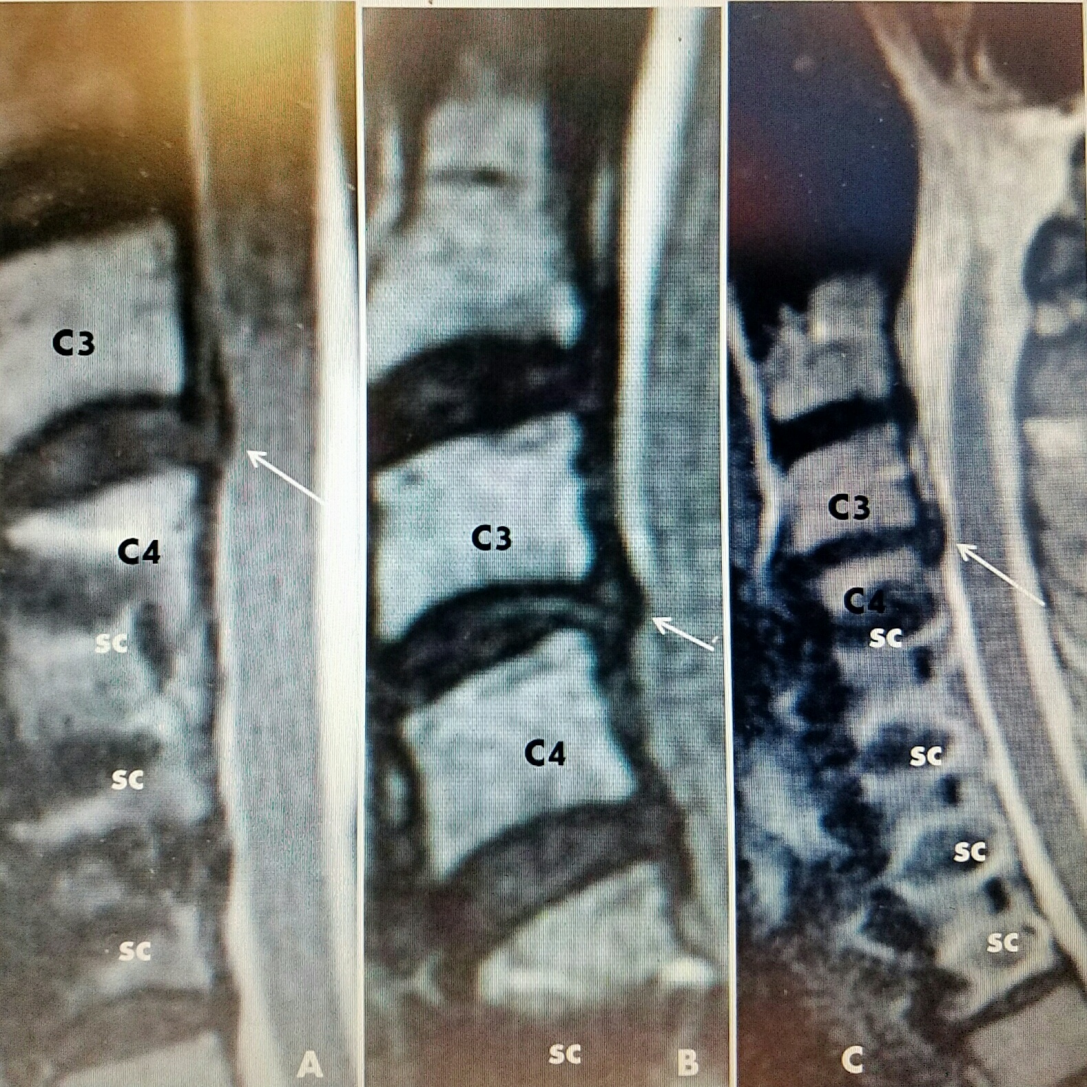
Sagittal MRI scans of three cases A: Case #1: previous C4-C6 fusion and plating with screws (sc), small C3-4 disc adjacent level (white arrow). B: Case #2: C5-C7 fusion and screws (sc) and small herniated disc C3-4 (white arrow). C: Case #3: Fusion with screws C4-C7 (sc) and slight disc narrowing and subligamentous herniation C3-4 (white arrow). MRI: Magnetic resonance imaging.
